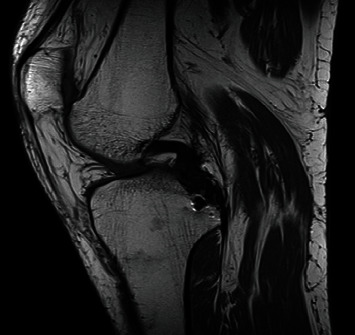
MRI at 2-year follow-up of Case 2. The LCP is inserted and continuous.
